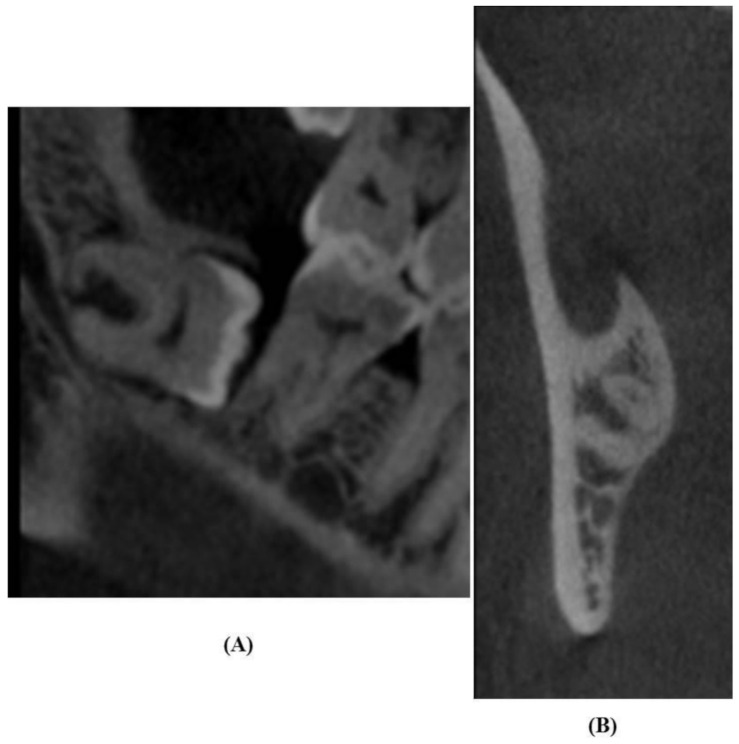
Panoramic images show diversion of mandibular canal (A), and buccally positioned inferior alveolar canal without cortication on CBCT images (B).
